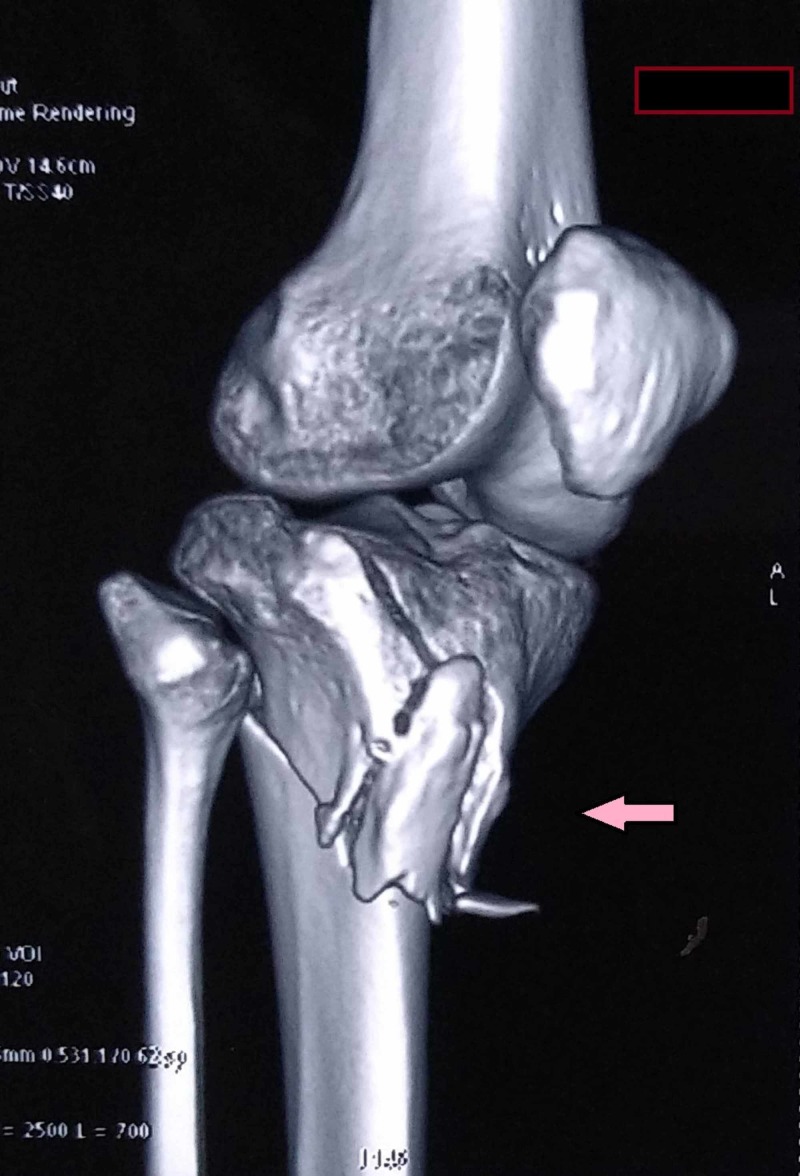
Three-dimensional computed tomography image showing a bicondylar fracture with tuberosity fracture (pink arrow)
